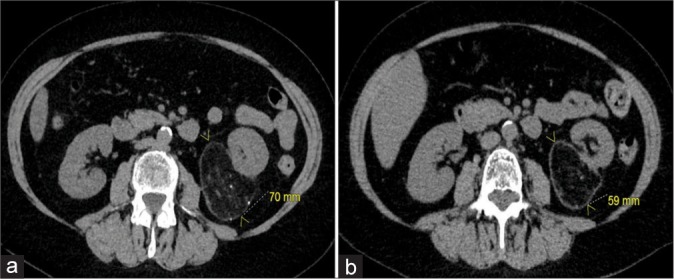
A 64-year-old woman with an incidental angiomyolipoma (AML). (a) Axial computed tomography (CT) of the abdomen and pelvis demonstrating the AML off the inferior pole of the left kidney measuring 70 mm. (b) Axial CT of the abdomen and pelvis at an 18-month follow-up showing shrinkage of the AML measuring 59 mm.
